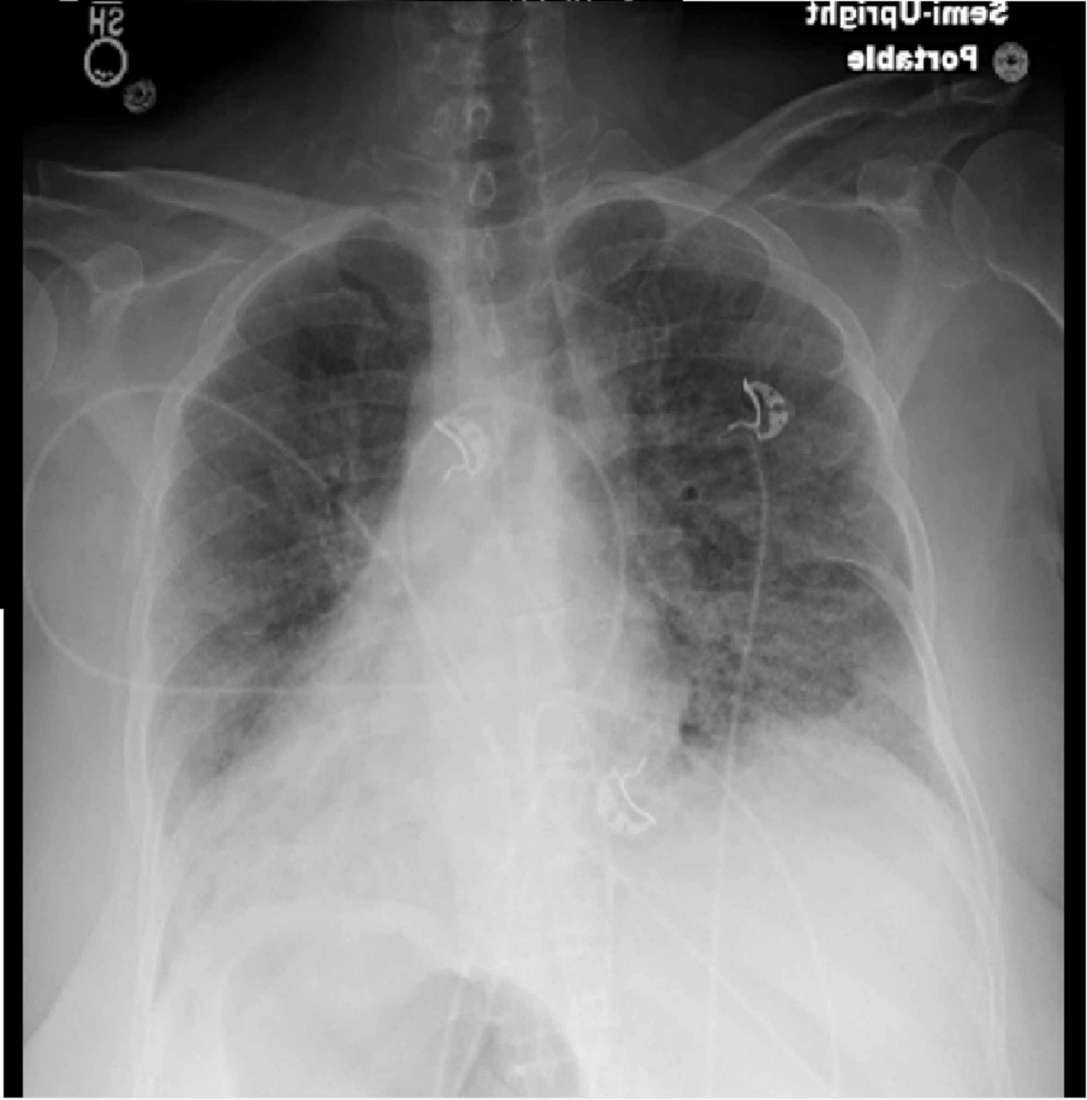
X-ray of the second day Hazy/patchy and interstitial opacity bilaterally overall increased could represent edema and/or infection, no large effusion or pneumothorax
